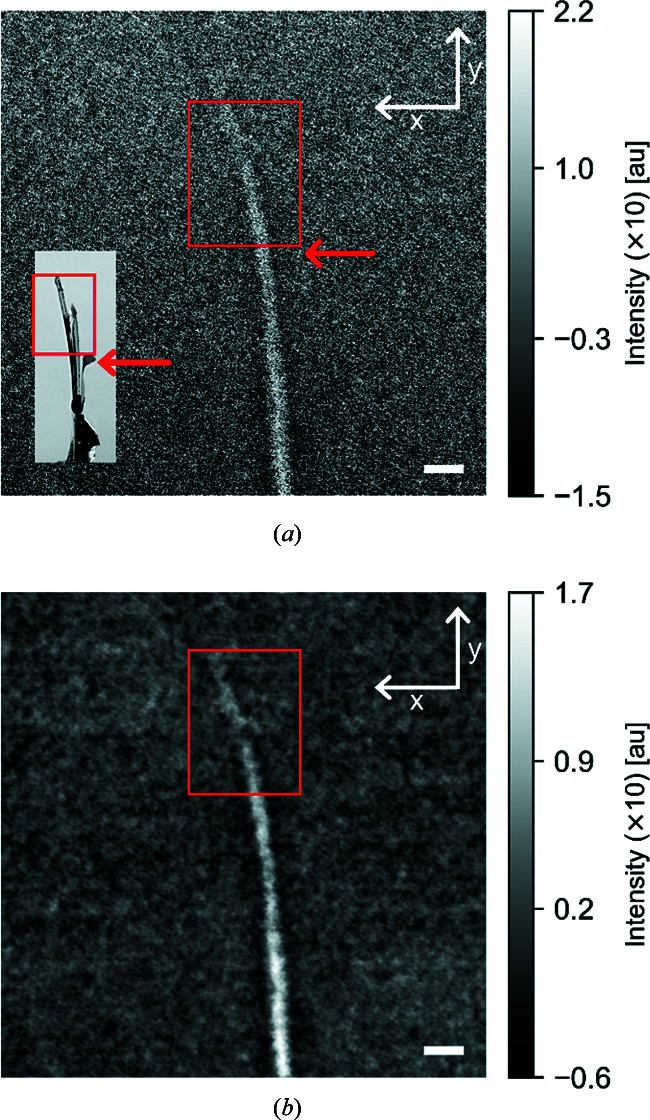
Neutron radiographs of the metal foil sample at a sample-to-detector distance Δ = 189 mm. The arrows point to the sample. Scale bars represent 1 mm. Radiographs (corrected as described in the *Experimental* section[Sec sec2]) are shown (*a*) without phase retrieval and (*b*) after phase retrieval. The inset in panel (*a*) shows a photograph of the metal foil sample. The red boxes indicate the same region in the photograph and radiographs. Note that the photograph is not recorded in the exact same orientation as the radiographs and the arrows do not point to exactly the same position in the sample and the signal.
